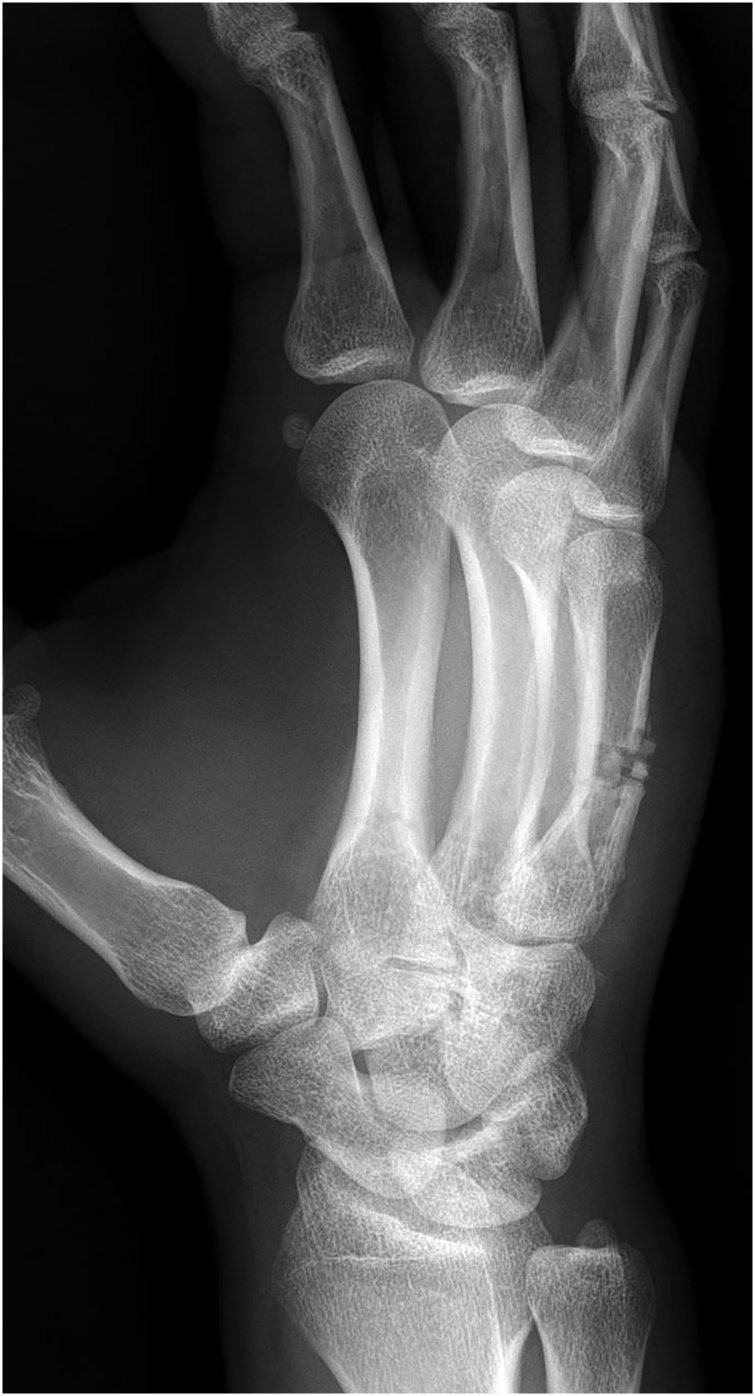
Iatrogenic fracture developed following failed K-wire tracks. Two weeks later, the patient came back with acute onset of pain and swelling at the 5^th^ metacarpal area. A radiograph of his hand was performed, which demonstrated a fracture through the metacarpal shaft where the K-wire had failed to enter the bone.
